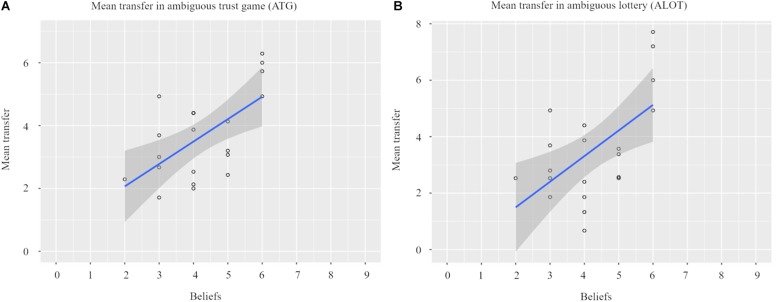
Elicited beliefs regarding receivers’ reciprocity influenced chosen transfer in the ambiguous trust game Panel (A). Based on individual beliefs, participants received a matching amount of winning colors in the ambiguous lottery. Participants used this information, given during instructions prior to the experiment in the MRI scanner, as transfer positively increased as a function of the amount of winning colors Panel (B).
